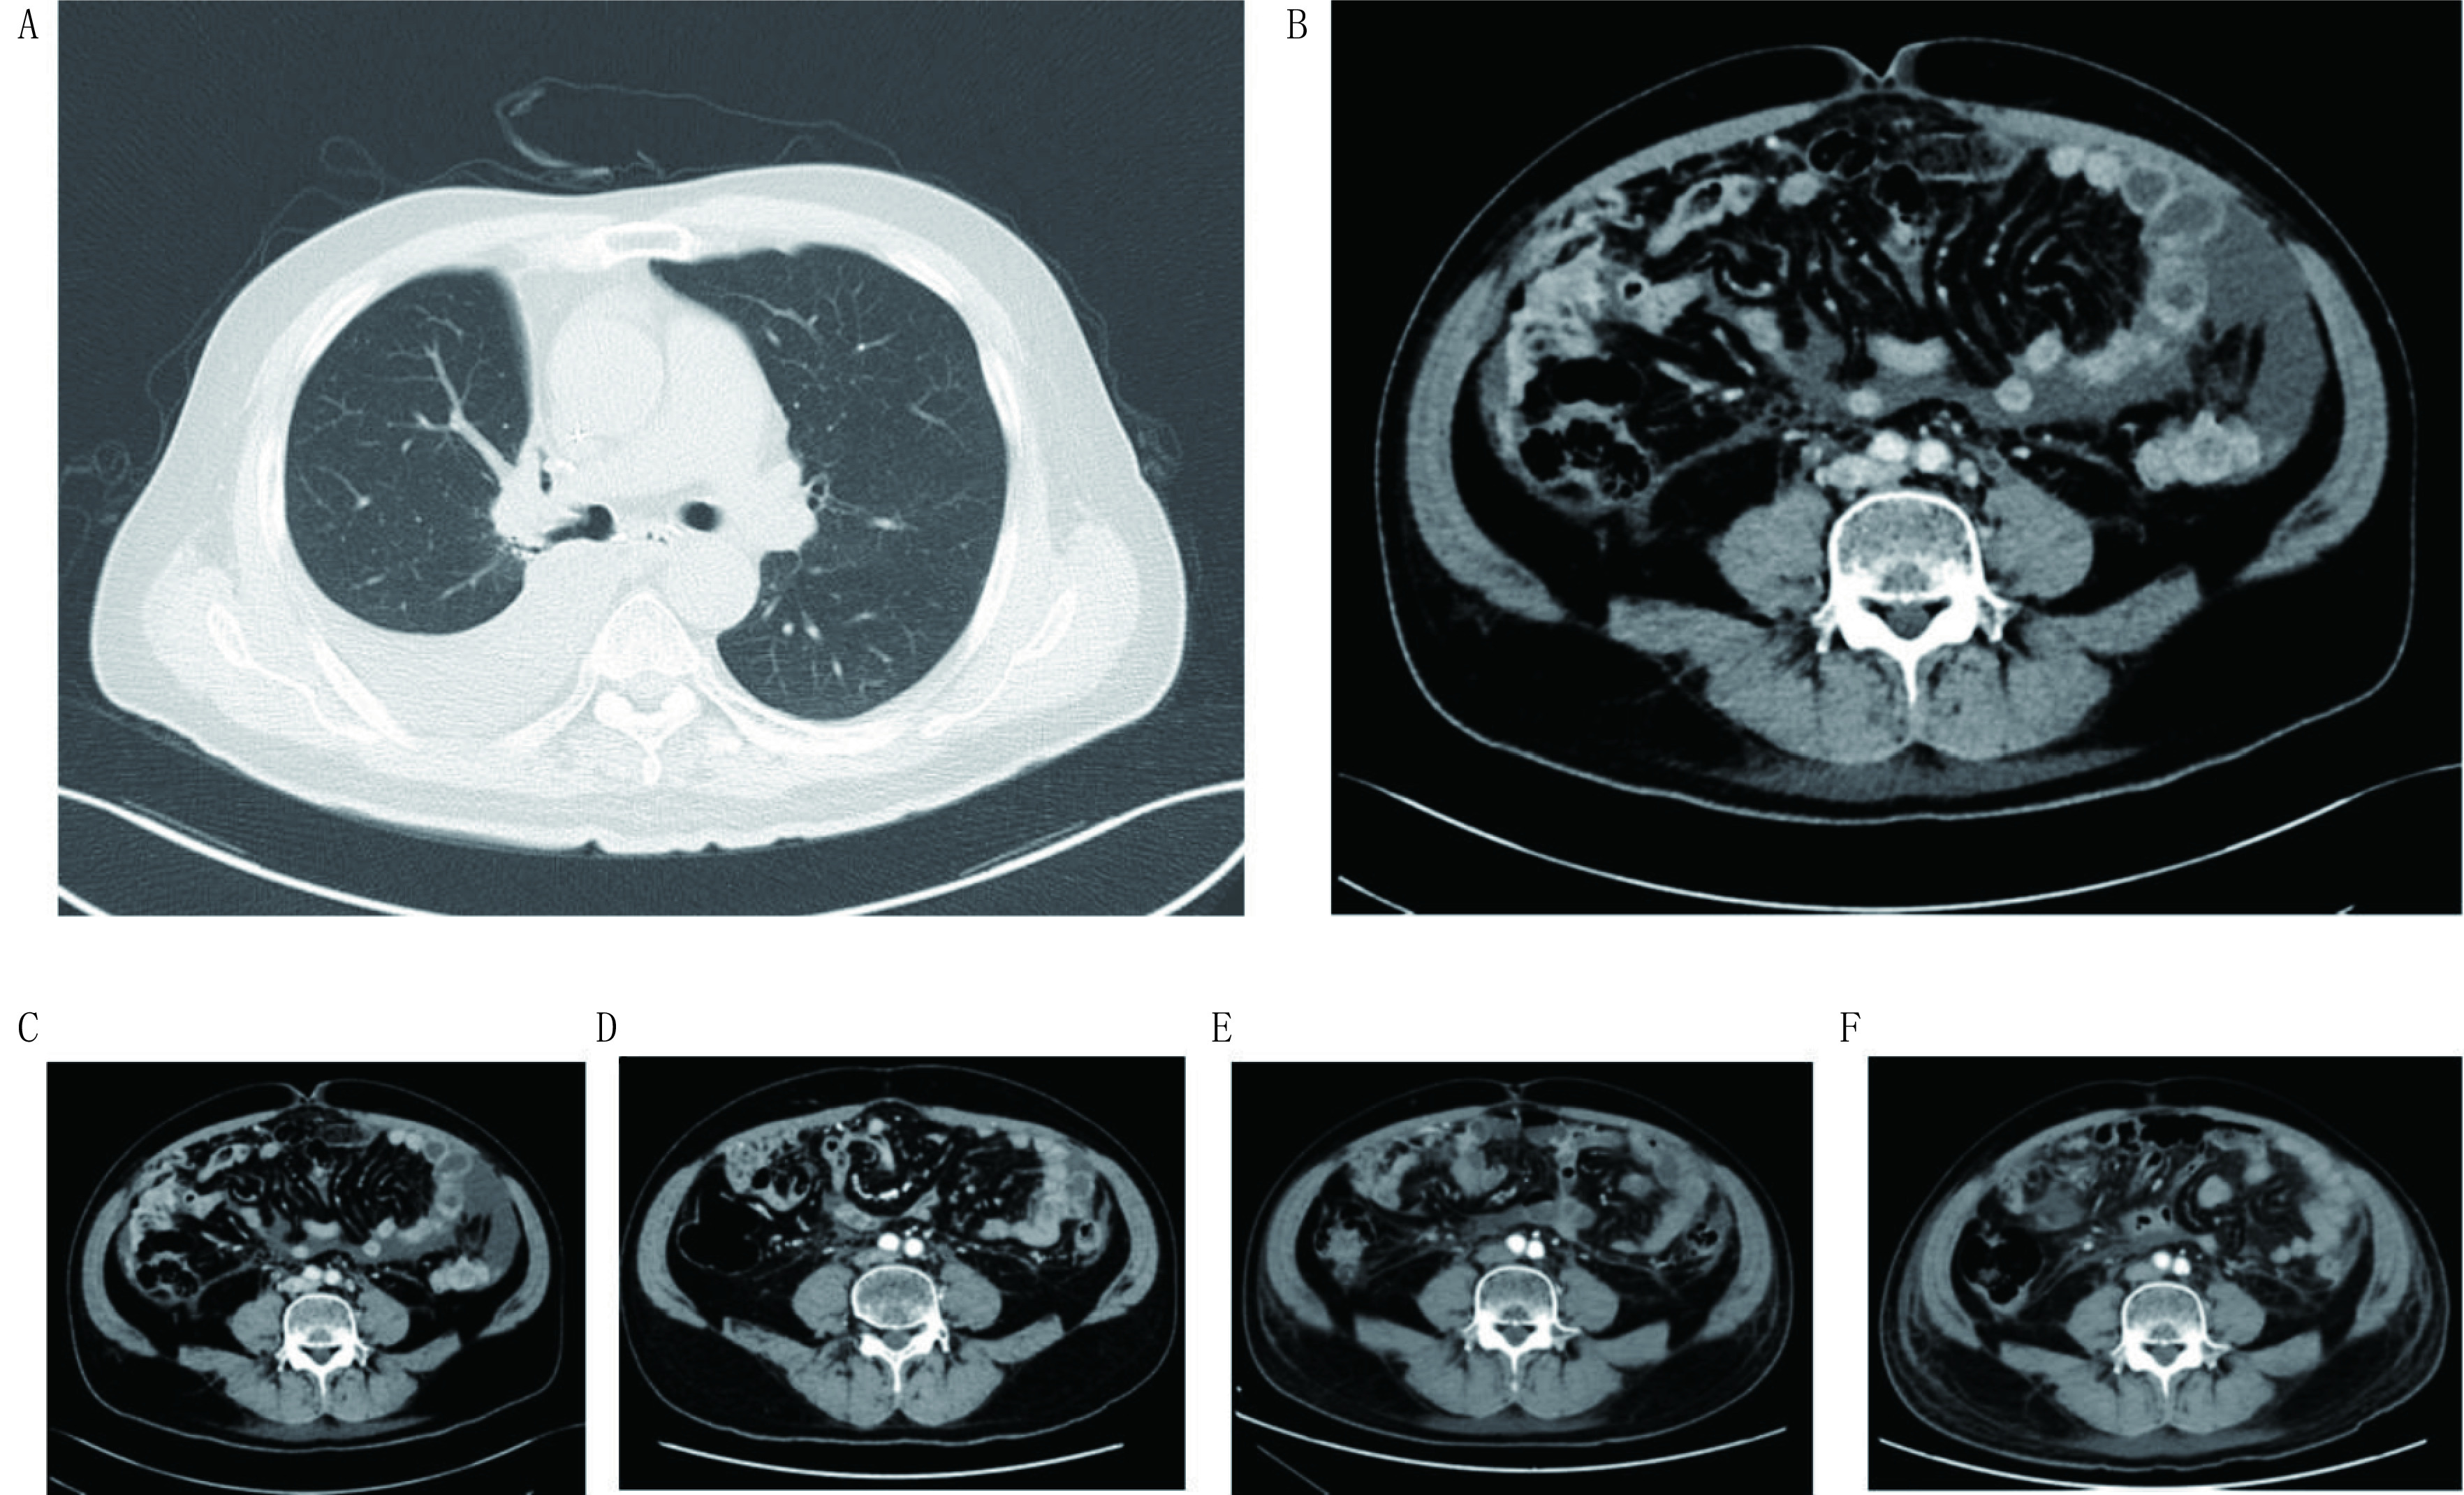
吉非替尼联合安罗替尼治疗患者1例。A：确诊PC胸部CT；B：确诊PC时腹部CT；C：确诊PC时；D：吉非替尼联合安罗替尼治疗1个月（腹水减少）；E：吉非替尼联合安罗替尼治疗3个月；F：确诊PC 6个月。 One patient with treatment of gefitinib and anlotnib. A: Chest CT image at PC diagnosis; B: Abdomen CT image at PC diagnosis; C: at PC diagnosis; D: 1 month after gefitinib plus anlotinib (only minimal ascites); E: 3 months after gefitinib plus anlotinib; F: 6 months after PC diagnosis.
